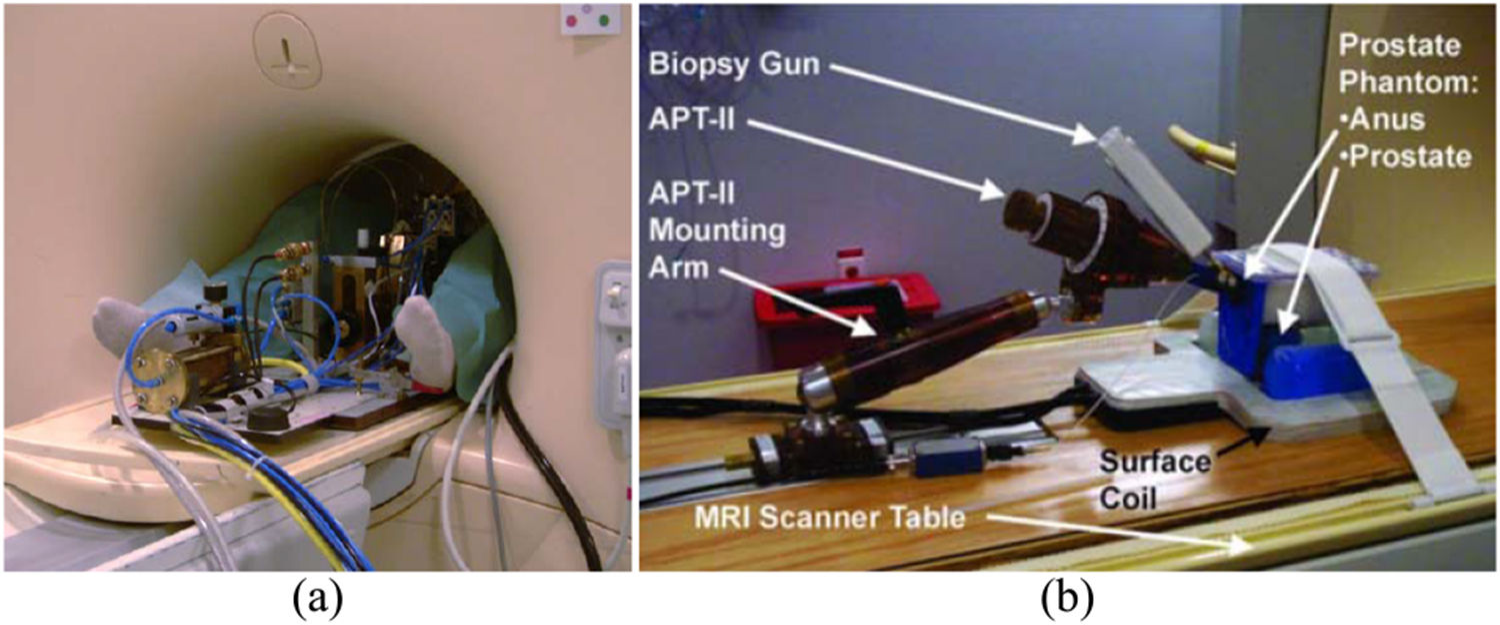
(a) Pneumatically and hydraulically driven robot for transperineal prostate interventions at the University Medical Center Utrecht [16]. (b) MRI-guided transrectal manipulator of the APT II system for transrectal needle access to the prostate was developed at Johns Hopkins University [14].
